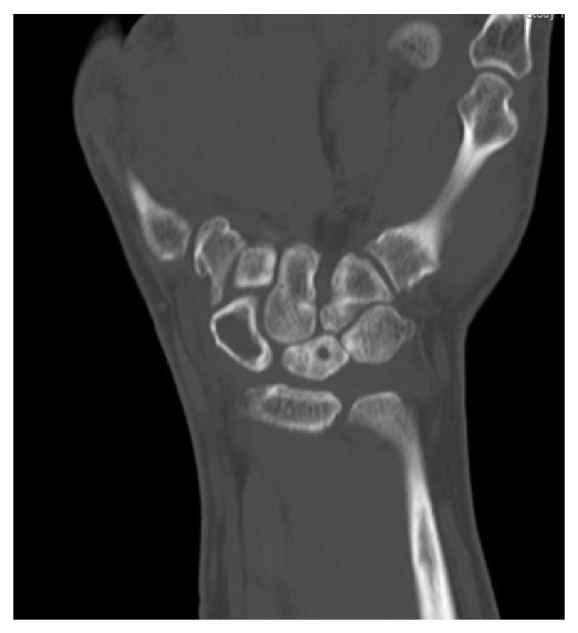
CT: giant interosseous ganglion of the scaphoid and sclerosis of the lunate.
